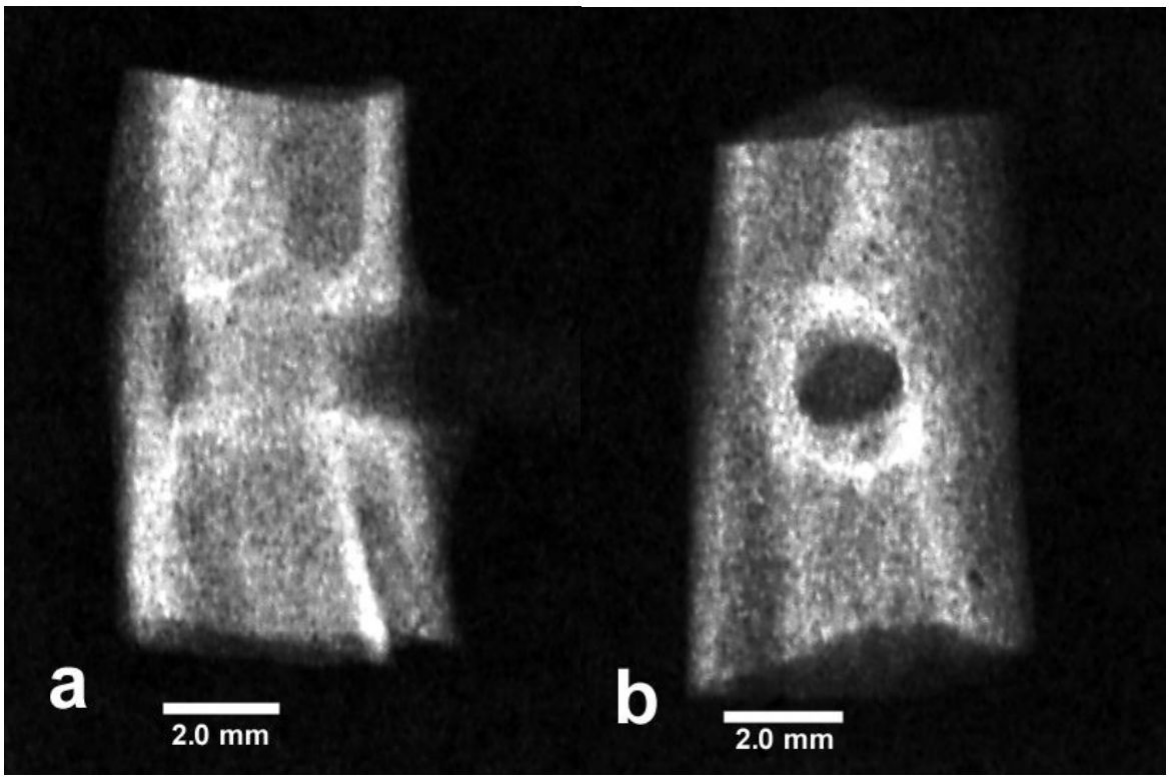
X-rays epoxy/carbon fiber PMC (a) Lateral view (b)
